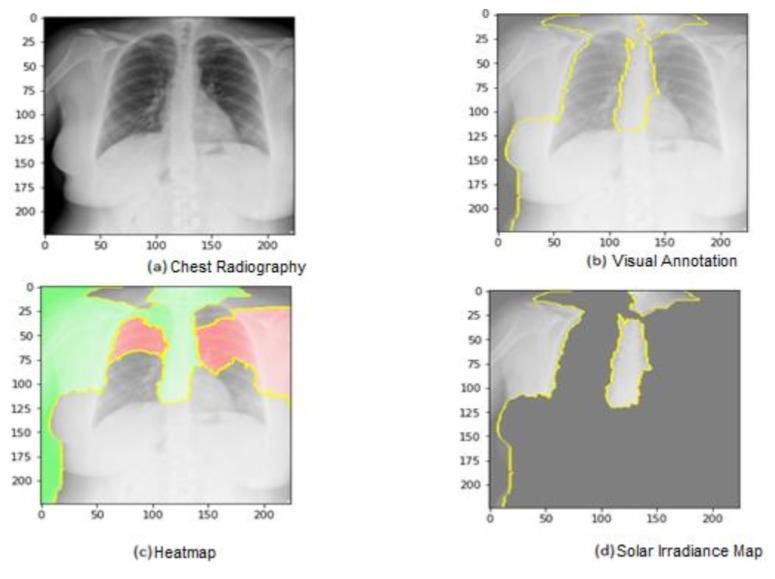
Explainability of X-ray images in the fifth dataset.
